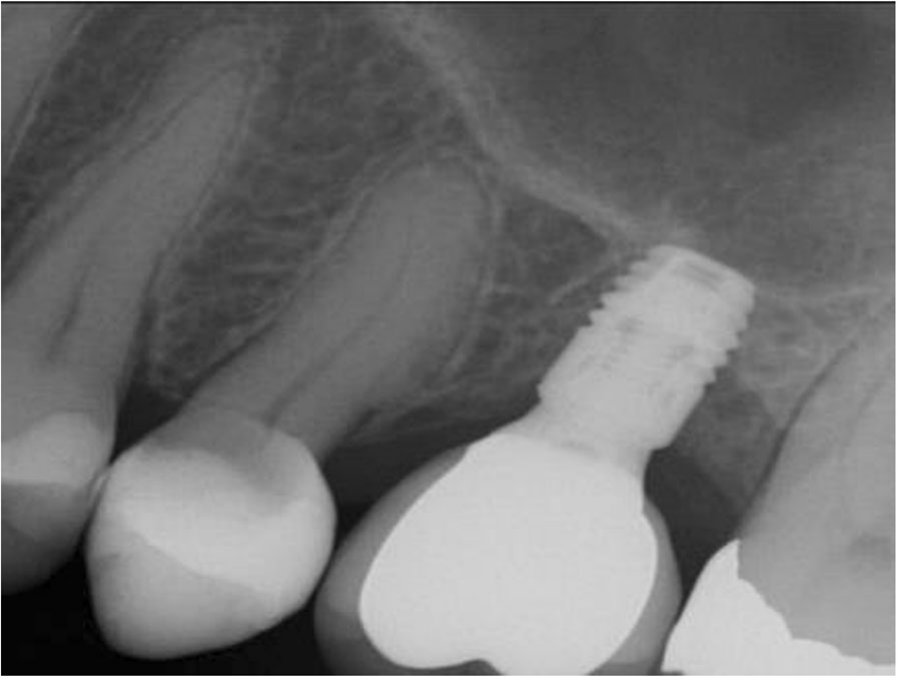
Periapical radiograph of a short implant one-year after functional loading
